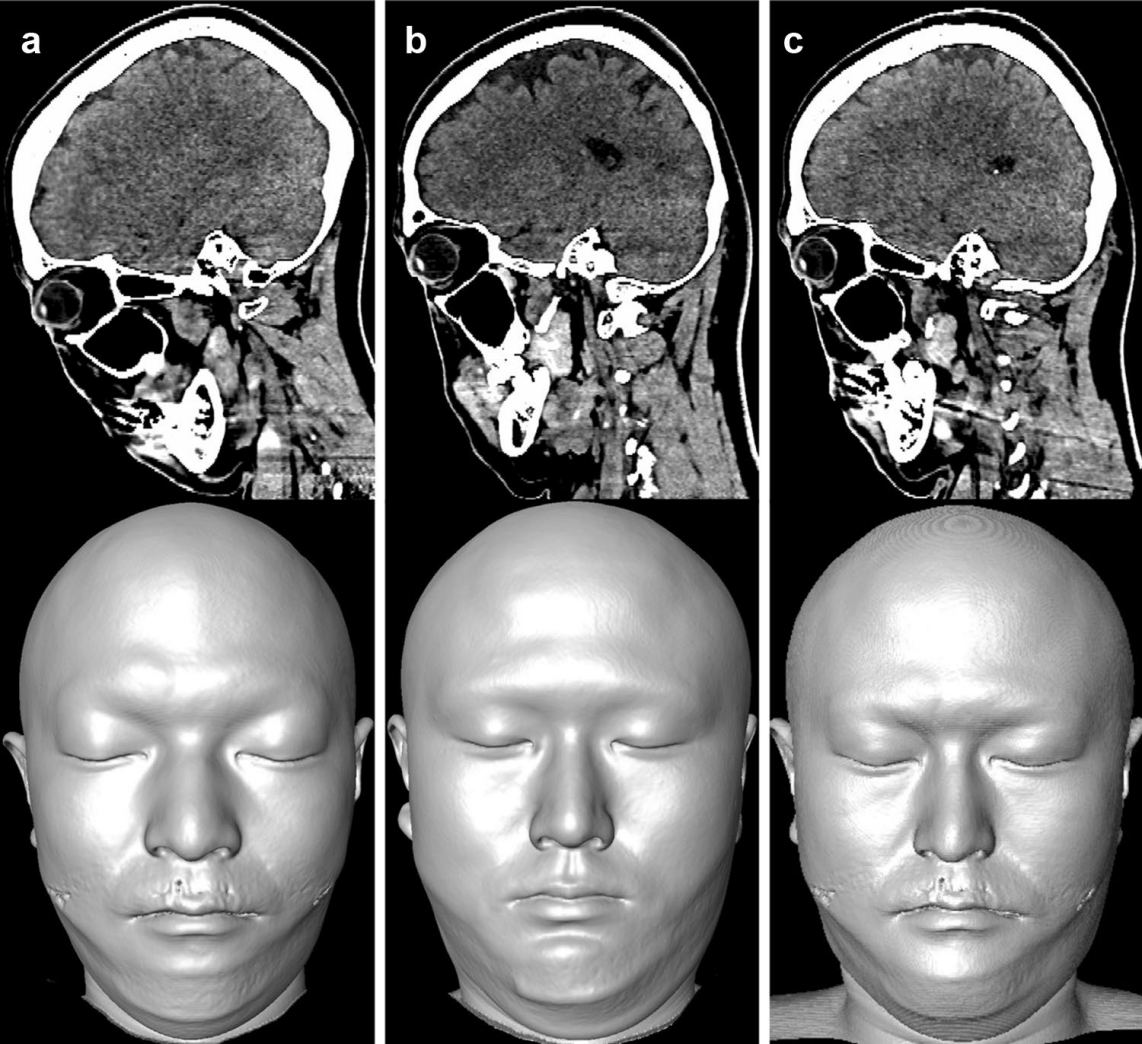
Deformation processing for the original image. Top: CT volume data, Bottom: reconstructed face models from above images. a original image, b reference image, and c deformed image. In all cases, the control points could be set, the reference images were selected, and the deformed images were created
